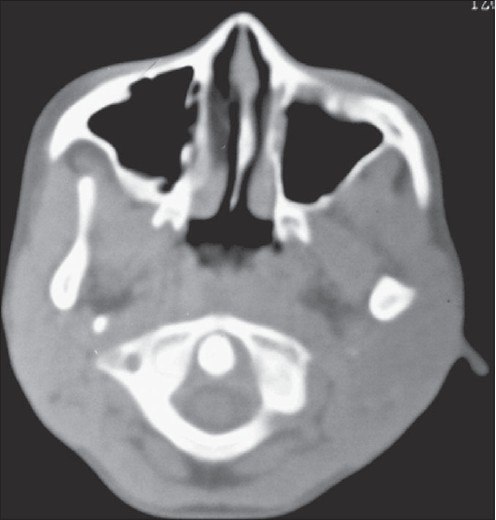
Computed tomography (bone window) showing swelling of the left parotid gland and no evidence of bony injury
